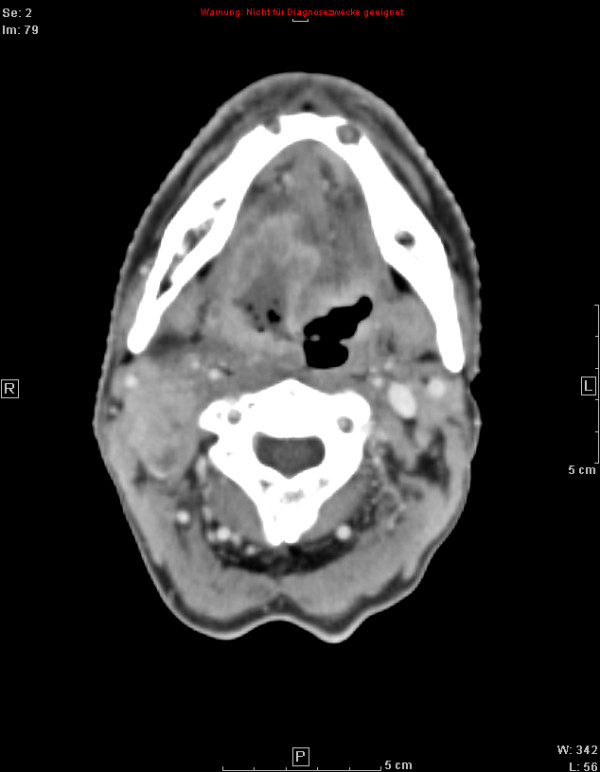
50 y old patient with oro-/hypopharyngeal carcinoma; planning CT.
